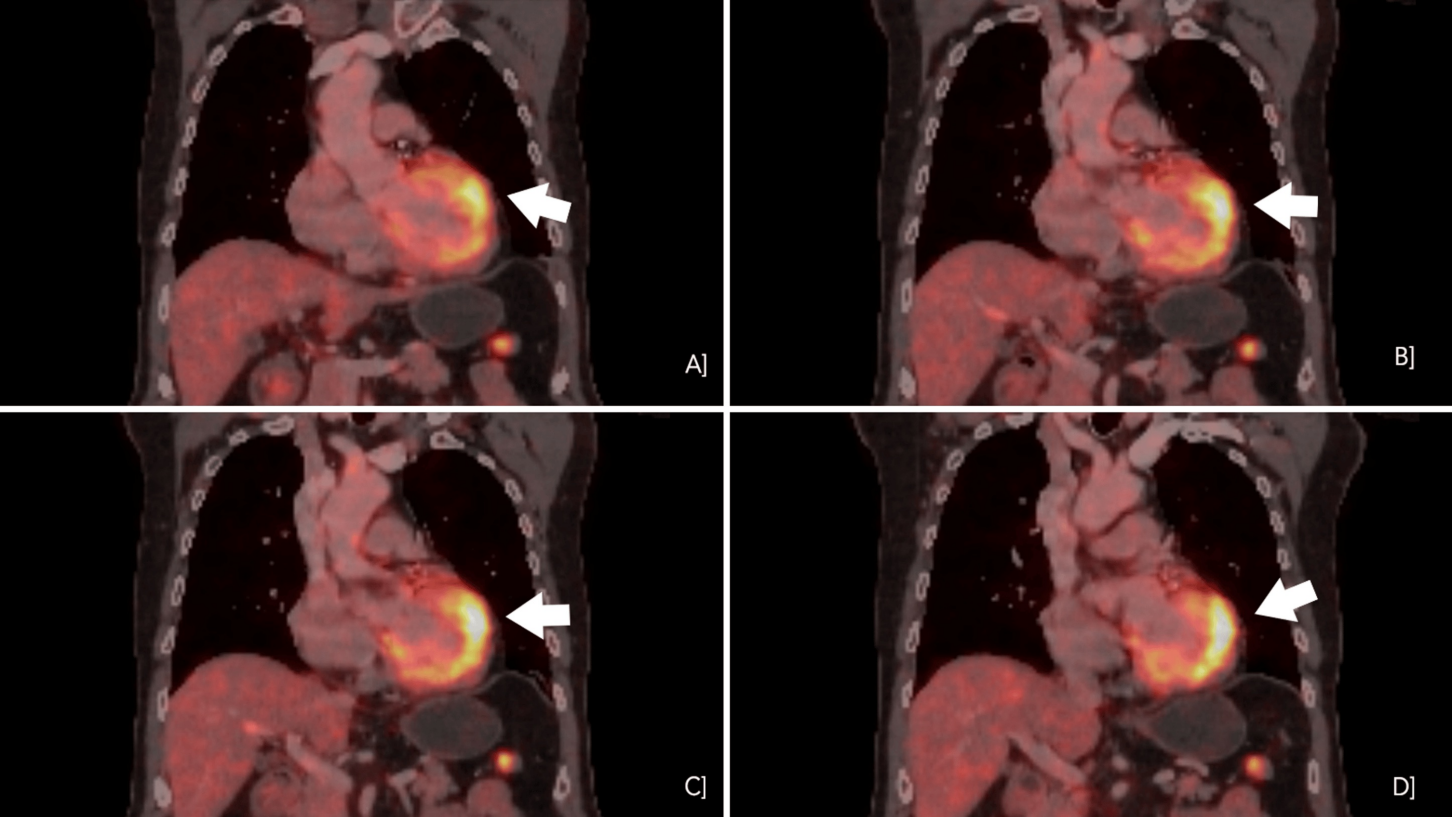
Coronal view of different slices of FDG PET-CT showing diffuse hypermetabolism of the anterolateral wall of left ventricle FDG PET-CT: Fluorodeoxyglucose positron emission tomography-computed tomography
